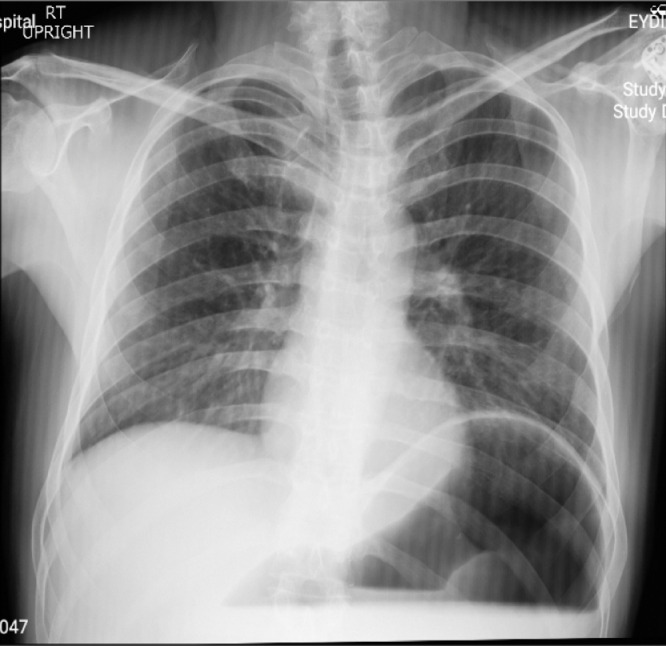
Upright chest X-ray indicates no evidence of pneumoperitoneum but the distended stomach is obvious along with an elevation of the left hemi-diaphragm.
